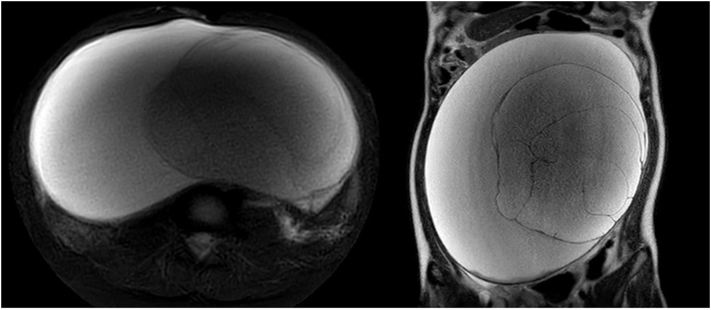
MRI findings. Predominantly cystic abdominal mass measuring 29 × 26 × 12 cm, originating from the left ovary.
